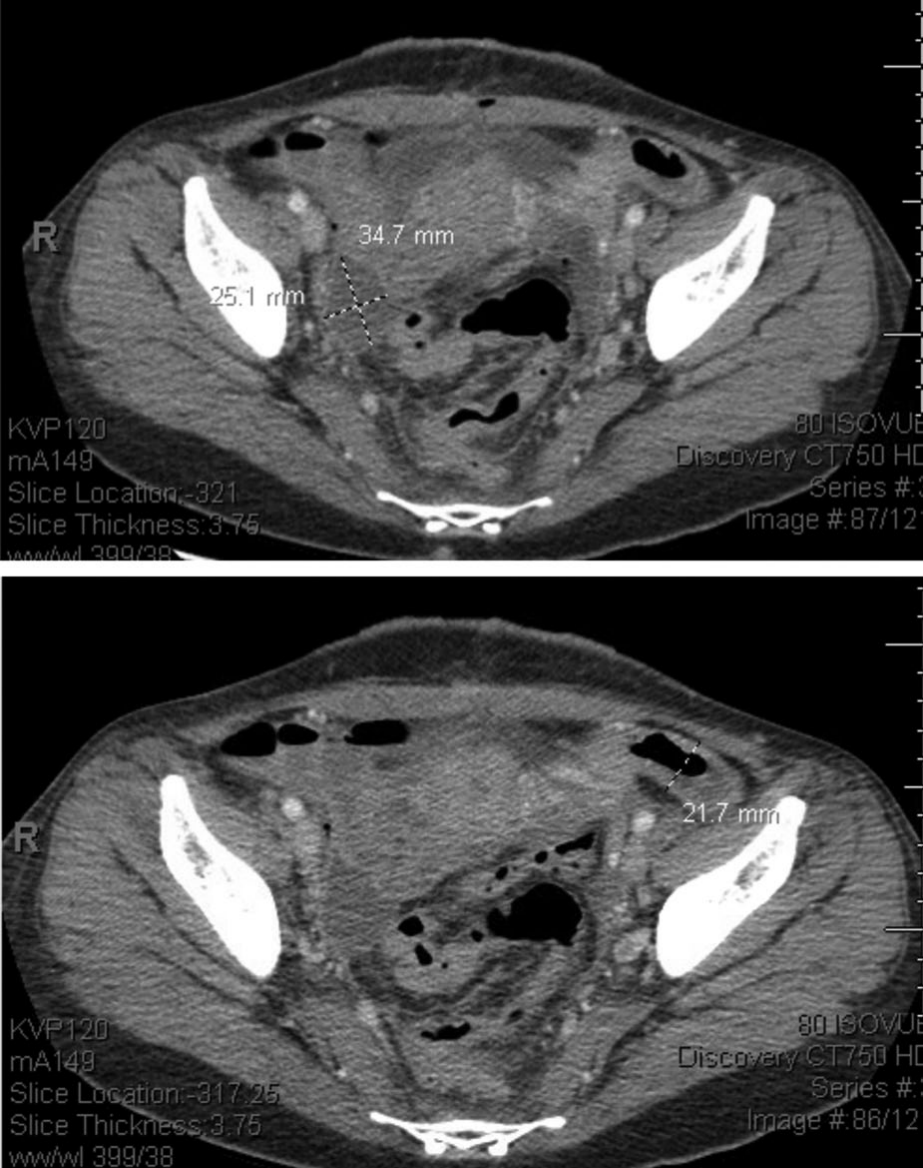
CT abdomen pelvis s/p exploratory laparotomy, right salpingo-oophorectomy with right adnexal mass removal, left adnexal mass drainage: right hemipelvic collection now measures 3.5 cm × 2.5 cm.
